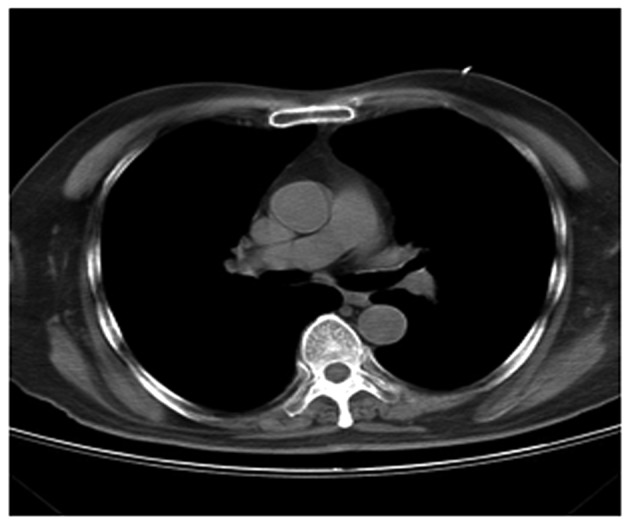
Cured tuberculosis following transdermal ultrasound treatment.
